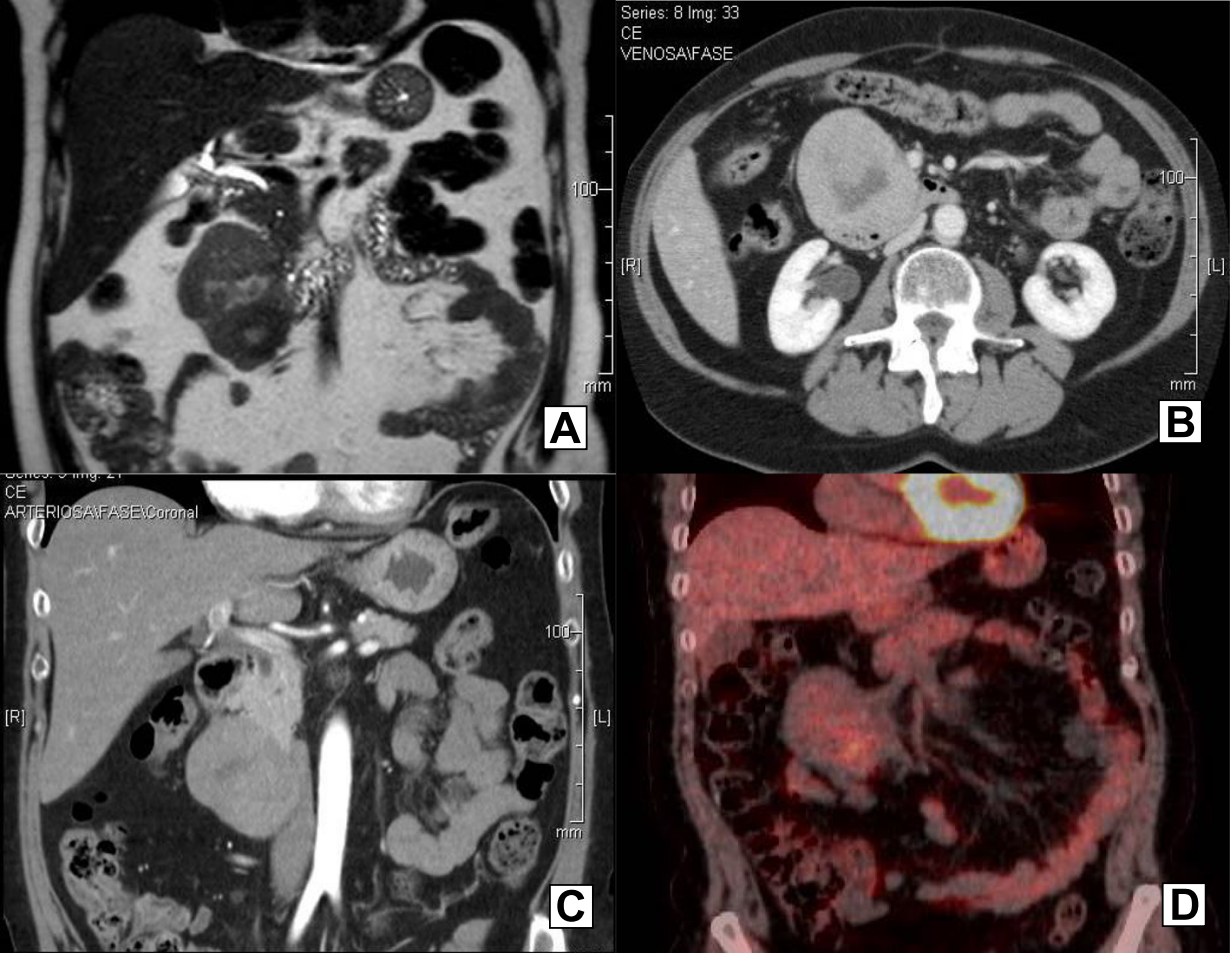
Pre-operative imaging. MRI scan: the dishomogeneous mass, arising from the pancreatic head (a). CT scan: no evidence of invasion of the surrounding tissues; CT venous phase: duodenal compression on the third duodenal part. (b) CT arterial phase: mass arising from the pancreatic head (c). ^18^F-FDG-PET-CT: moderate tracer uptake by the pancreatic lesion (d)
